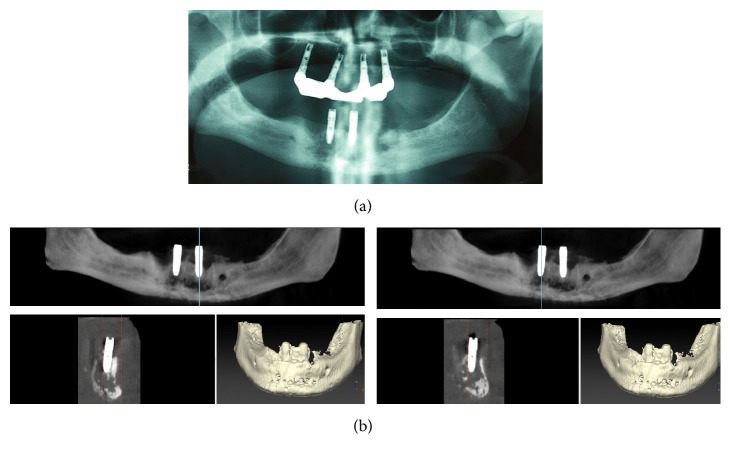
(a) Panoramic radiograph showing bone osteolytic lesions without defined borders after the second-stage surgery. (b) CBCT urgently performed showing osteolysis, with lingual and buccal cortical interruption.
